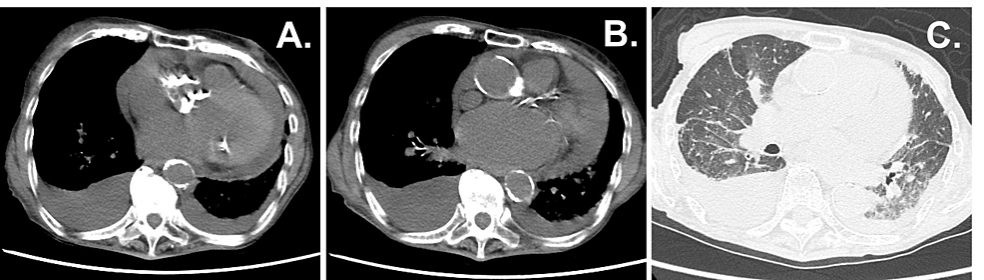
Plain CT of the patient (day 1) Panels A and B show cardiac dilatation, pericardial and bilateral pleural effusion, and severe calcification of the aortic valve, mitral valve, aorta, and coronary arteries. Panel C shows pulmonary congestion.
